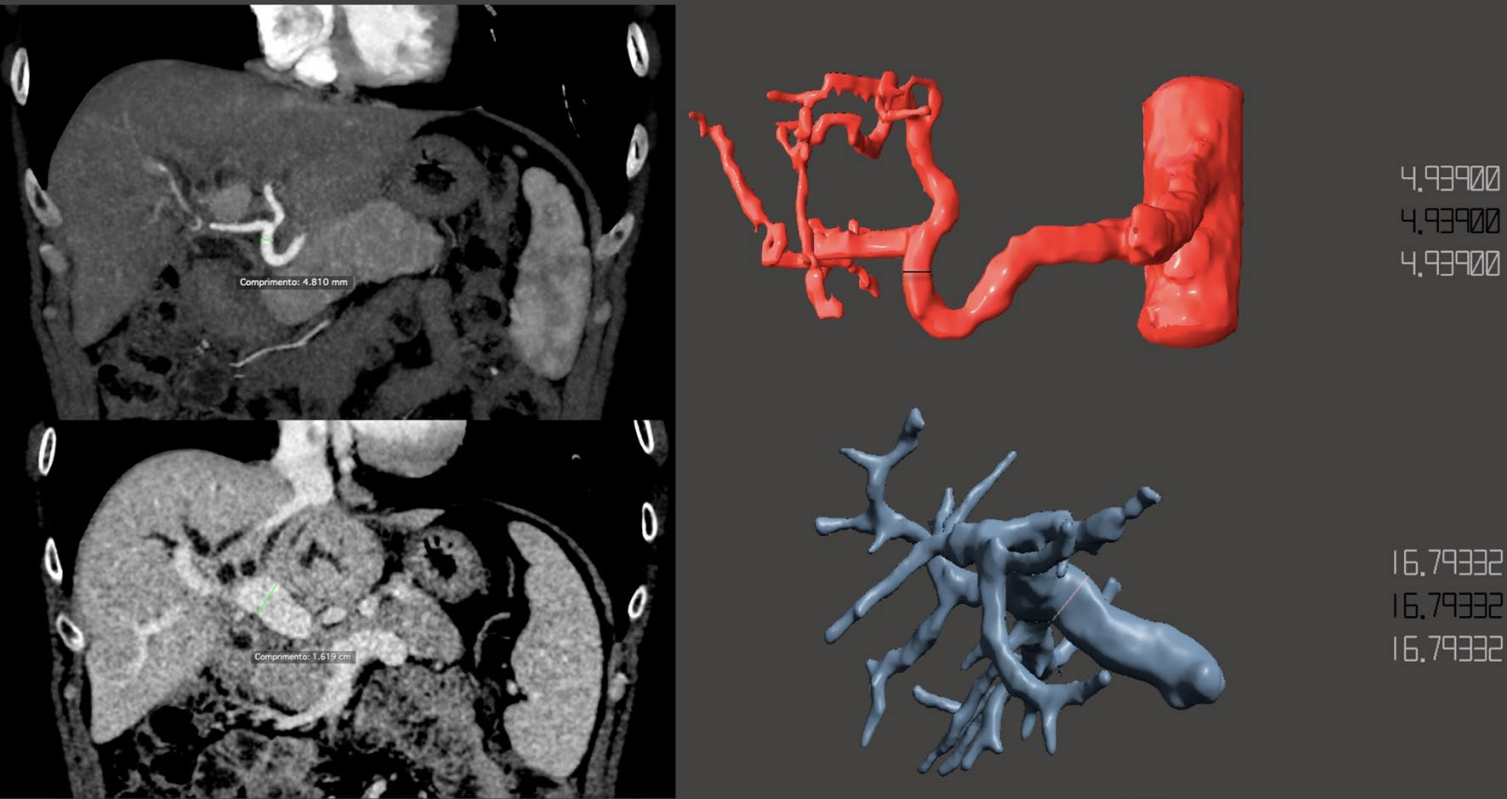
Measurements of the hepatic artery (top row) and main portal vein (bottom row) in both CT and STL
